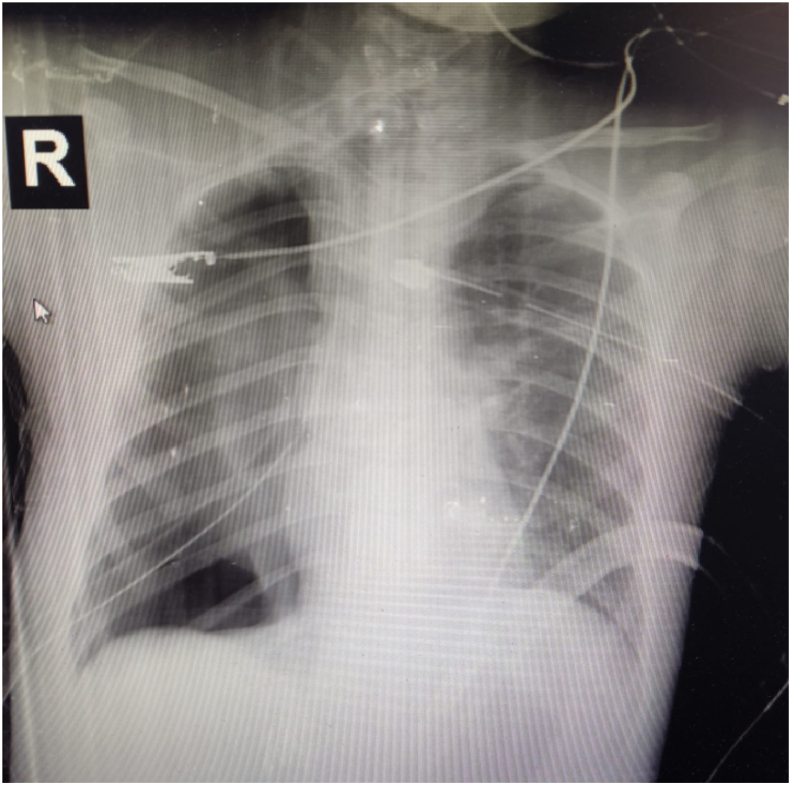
Supine radiograph shows a large right-sided pneumothorax after primary repair of right main bronchus avulsion despite use of chest drains.
